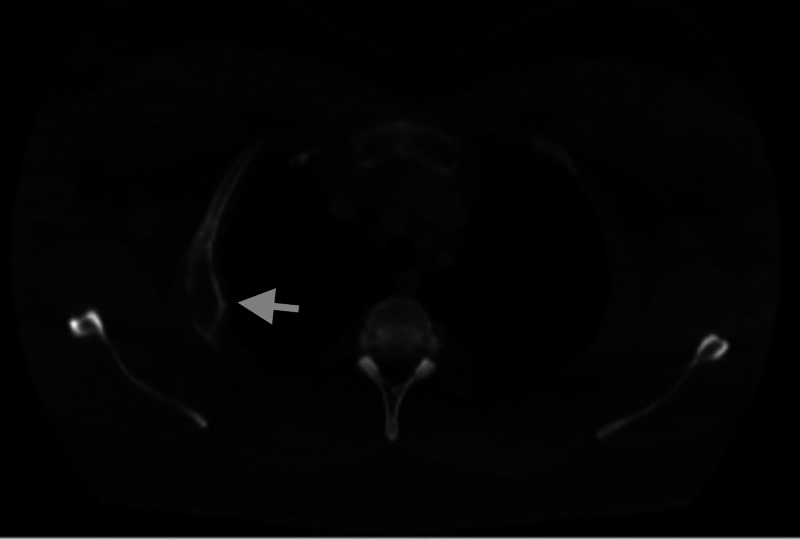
CT scan demonstrating expansile lytic lesion in the second right rib
